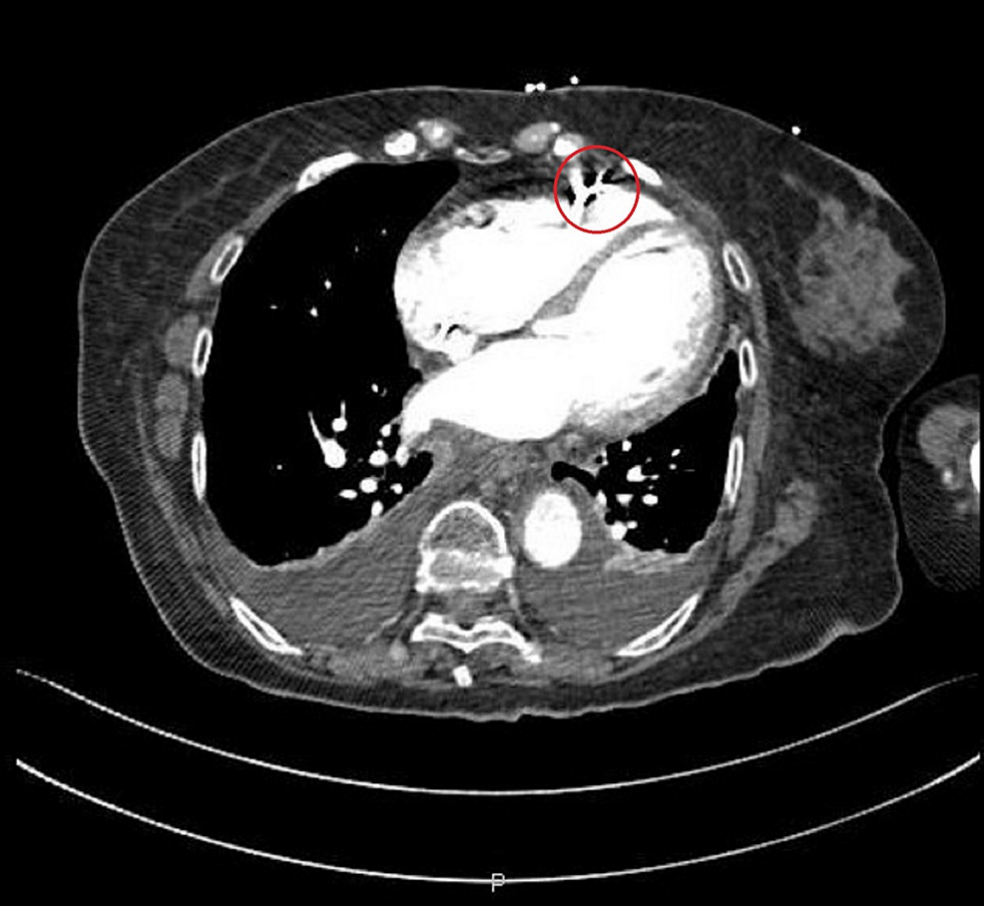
Thoracic CT scan revealing dislodgment of the right ventricular lead and bilateral pleural effusion.
